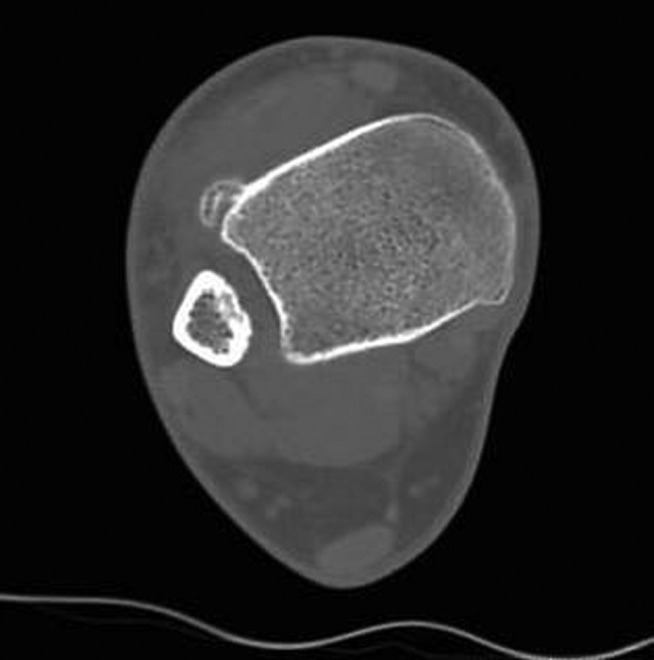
CT image of a Nora lesion arising from the antero-lateral surface of the tibia.
